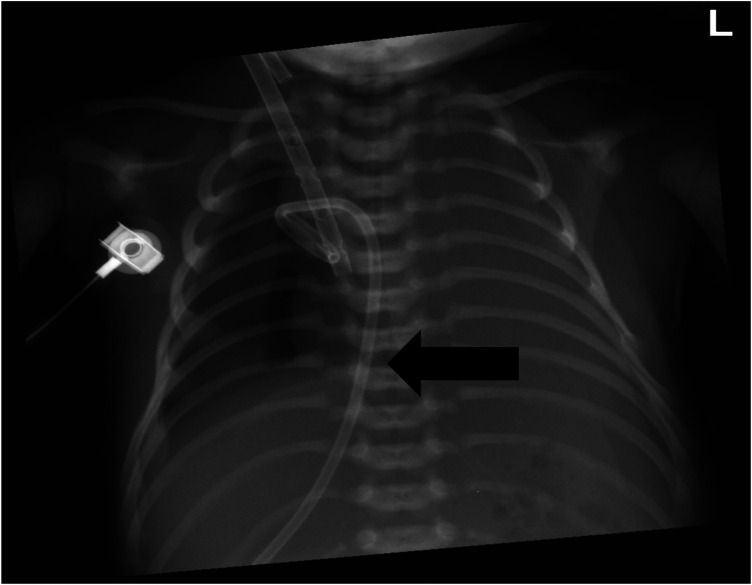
Chest x-ray showing partial improvement of right pneumothorax.
